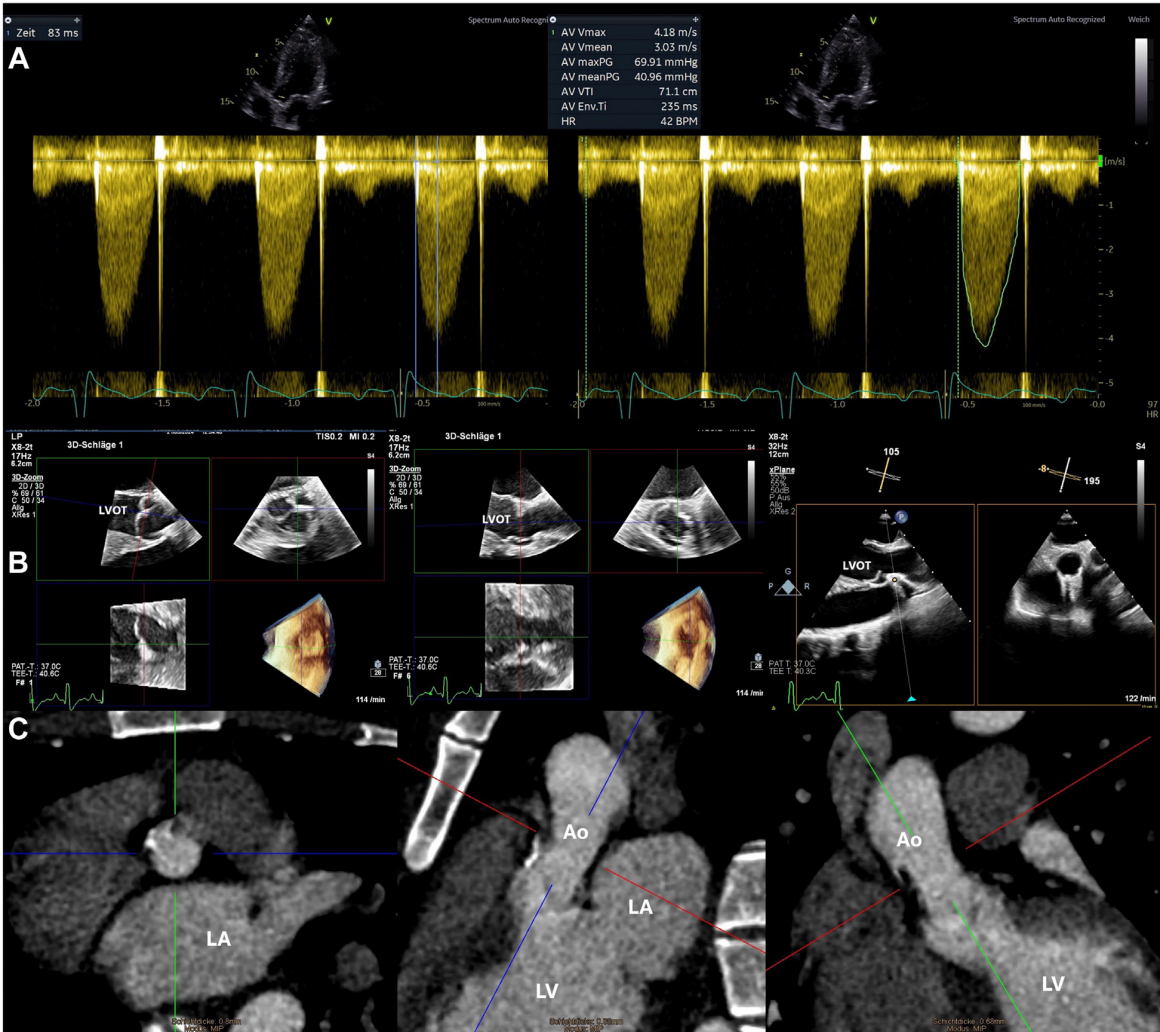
Echocardiographic and Computed Tomographic Images (A) Echocardiography-derived pressure gradients across the aortic valve including acceleration time. (B and C) Transesophageal echocardiography and computed tomography of the aortic valve and ascending aorta. Ao = aorta; LA = left atrium; LV = left ventricle; LVOT = left ventricular outflow tract.
